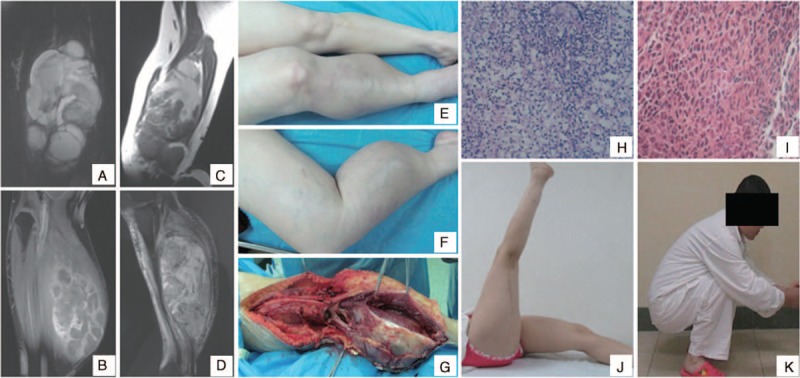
(A,B) Magnetic resonance imaging (MRI) before chemotherapy, the large soft tissue tumor in right lower limb, from posterior thigh to posterior lower leg, its boundary was not clear. (C,D) MRI after 2 cycles of chemotherapy, the tumor volume reduced, and its boundary was clear. (E,F) Appearance before operation. The tumor was large, and the en-bloc resection was difficult. (G) There was clear boundary of the tumor in operation, and its membrane integrity. (H) Biopsy pathology: small cell malignant tumor, primitive neuroectodermal tumor (HE × 200). (I) Postoperative pathology: the tumor cells exhibited necrosis (HE × 200). (J,K) Postoperative appearance after 3 years. The right lower limb function is good, and the patient can squat, jump, and run.
